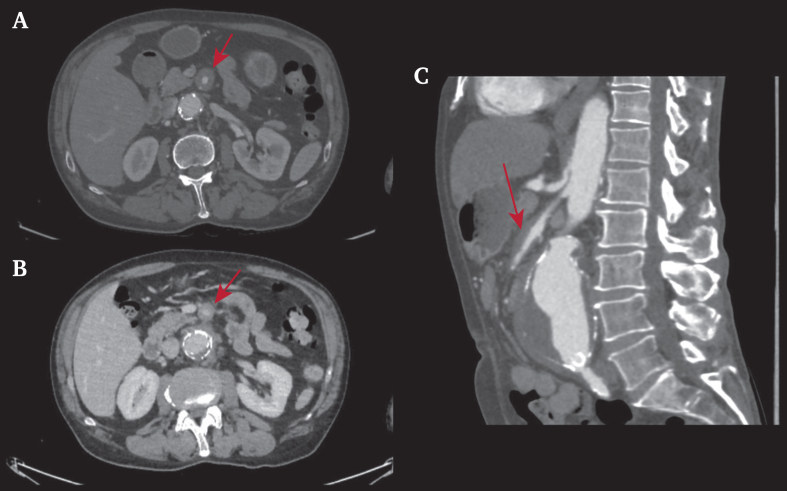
Computed tomography angiography The red arrows indicate the inflammatory segment of the superior mesenteric artery.
